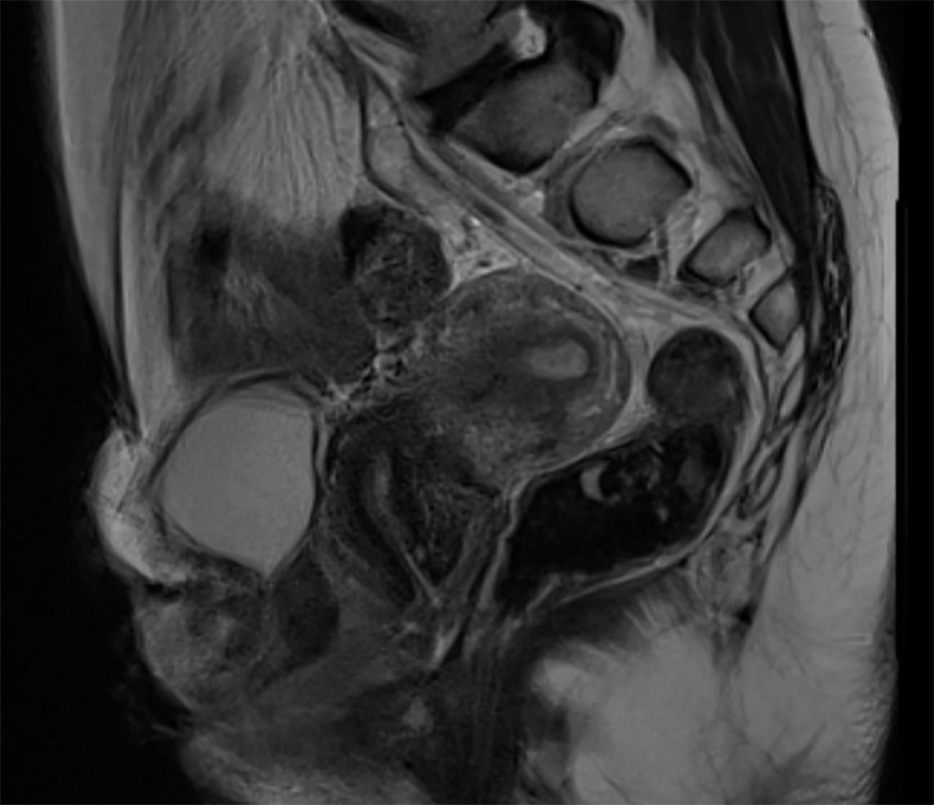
MRI of the abdomen/pelvis of the patient. Sagittal view from the patient. Anteriorly the non-exstrophic bladder is visible, uterus and rectum are clearly visible.
